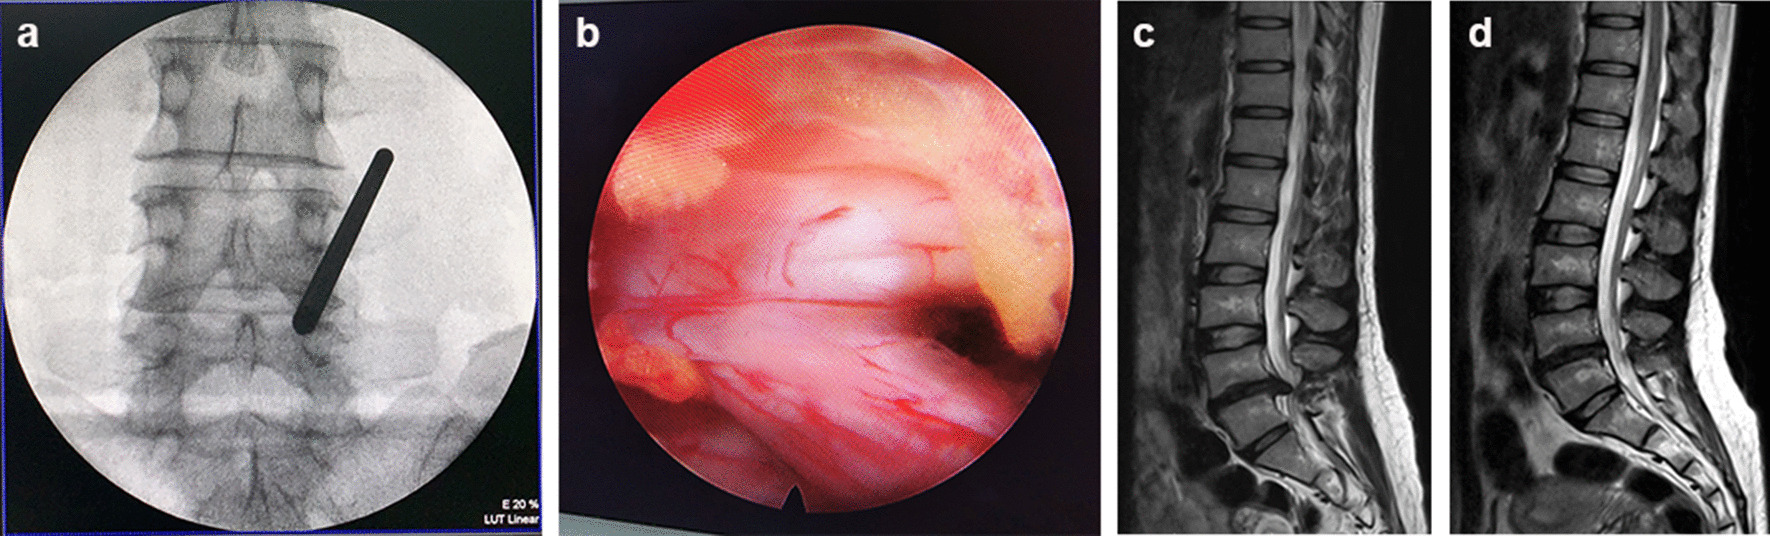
EILD. a Preoperative fluoroscopy localization, b macroscopic observation of nerve root under endoscope after operation, c preoperative MRI sagittal image, d post-operative MRI sagittal image
